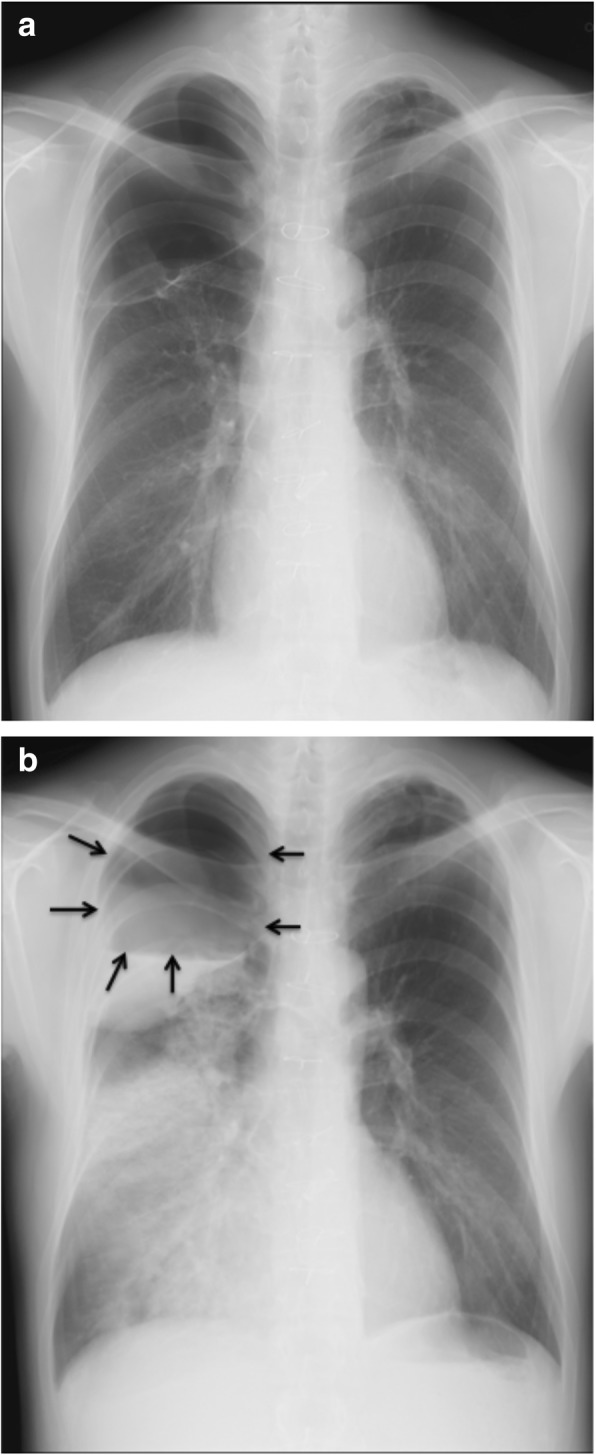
Chest X-ray showing an apical giant bleb in the right lung before a massive haemoptysis (a). There was an air fluid as an accumulation of blood in the bleb and a large consolidation in the middle and lower lung after bleeding (b). The arrows indicate the margin of the pulmonary cyst and its fluid level (b)
